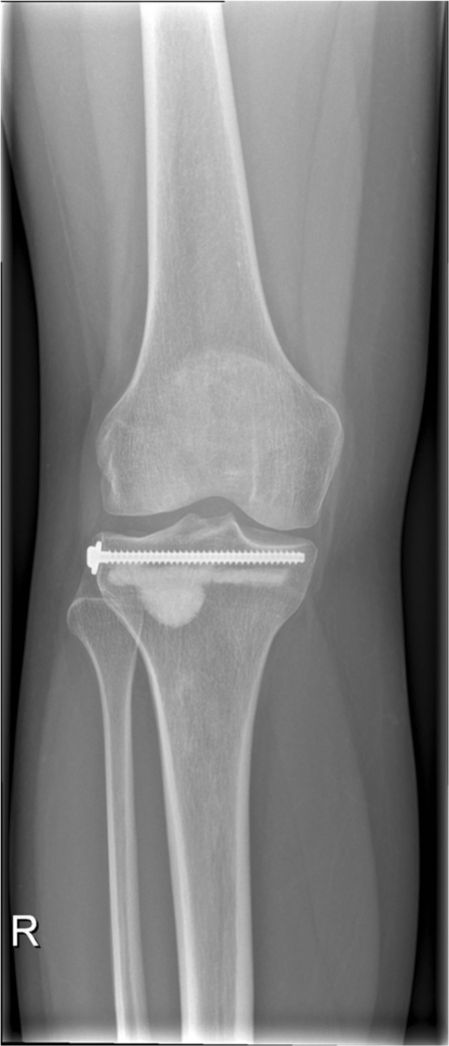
Anteroposterior X-ray view of a successful cement-augmented tibioplasty of a tibial plateau fracture (Schatzker II)
